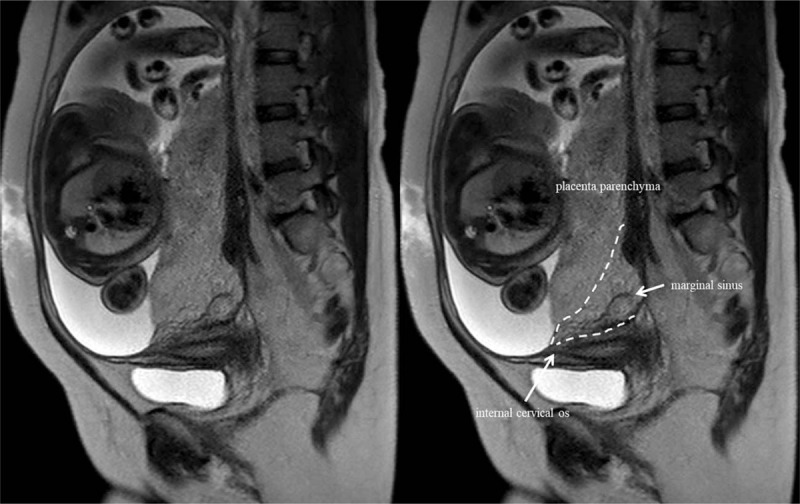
Representative magnetic resonance imaging of marginal sinus placenta previa (sagittal T2-weighted imaging).
